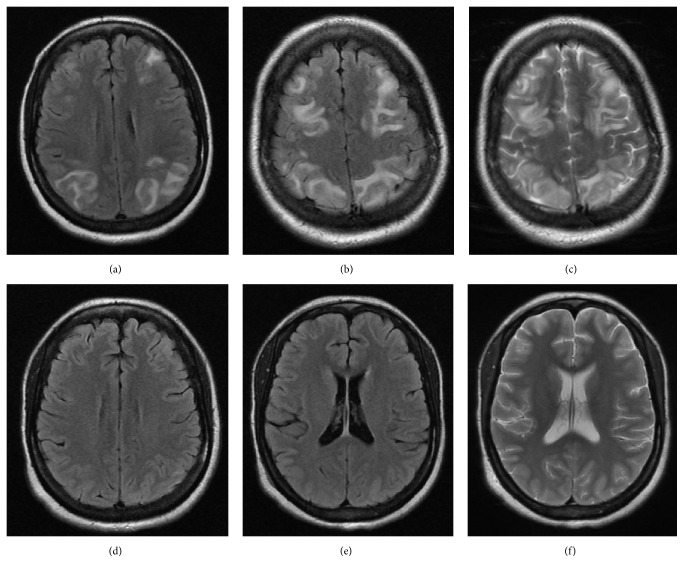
The initial MRI (images (a)–(c)) showed typical holohemispheric involvement: axial view in FLAIR (see (a), (b)) and T2-weighted (see (c)) images with extensive areas of high signal intensity in the subcortical white matter of both parietooccipital regions, including frontal lobes. Second MRI (images (d)–(f)) performed 2 weeks later with no signal abnormality within the brain parenchyma on FLAIR (see (d), (e)) and T2-weighted (see (f)) images.
